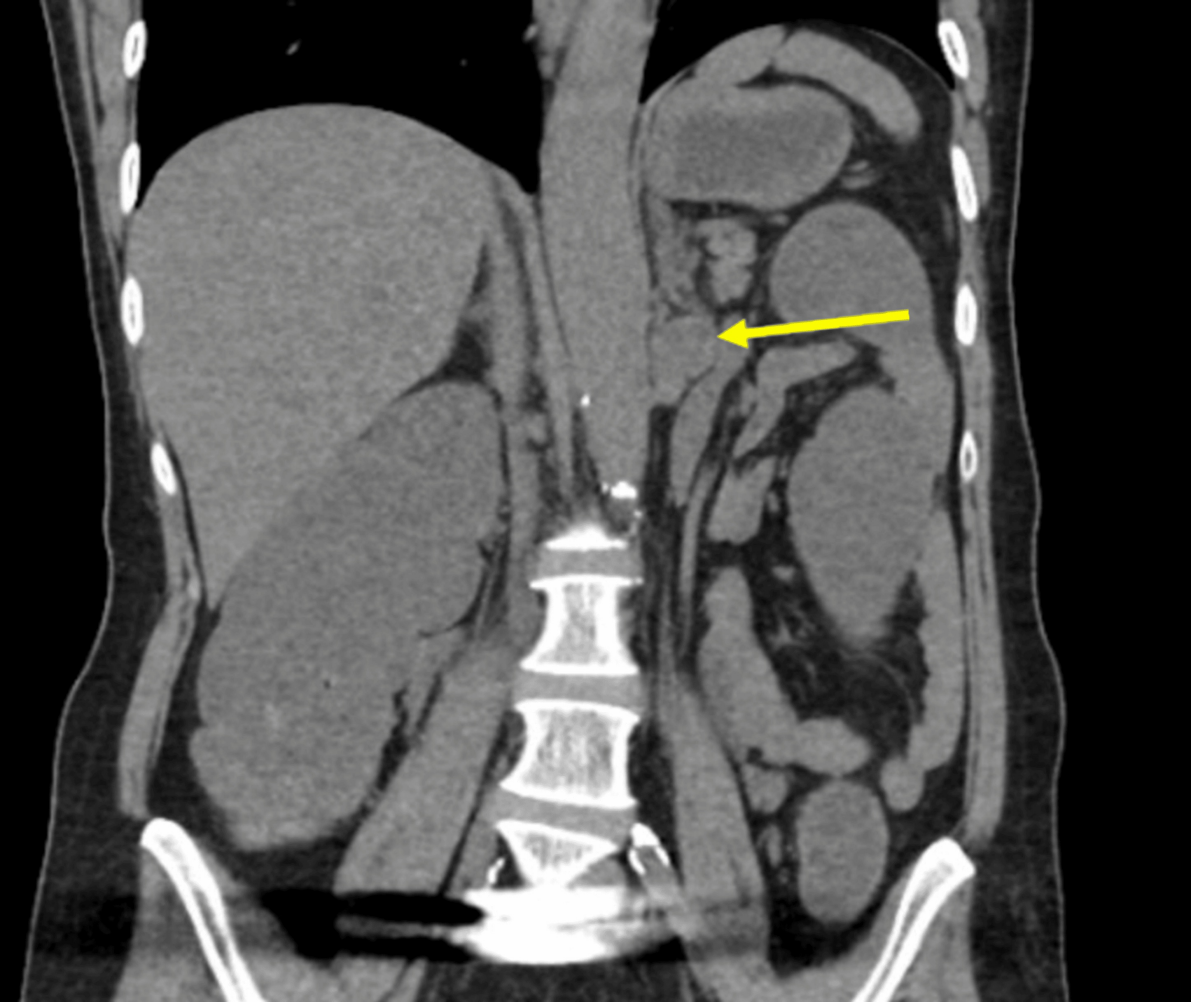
Retroperitoneal mass (yellow arrow) seen on non-contrast CT in September of 2021 CT: computed tomography
